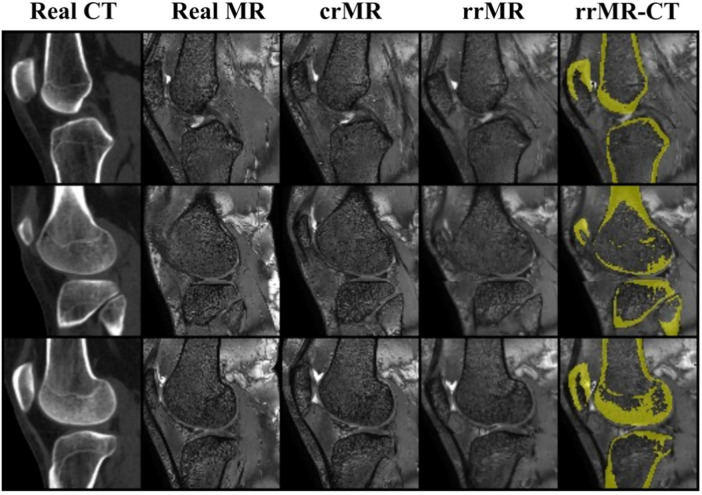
Comparisons of different registration methods. Each row shows the synthesis results of a sampled slice from the test dataset. From left to right, the columns are sequentially real MR images, real CT images, coarsely-registered MR (crMR) images by ANTs (rigid only) (32), refined-registered MR images (rrMR), and the visualization of registration performance between rrMR and real CT. In the visualization images, the high signals on the registered CT images are colored yellow and overlaid onto the corresponding rrMR images. The visualization shows a satisfied structural alignment situation of rrMR and CT images.
